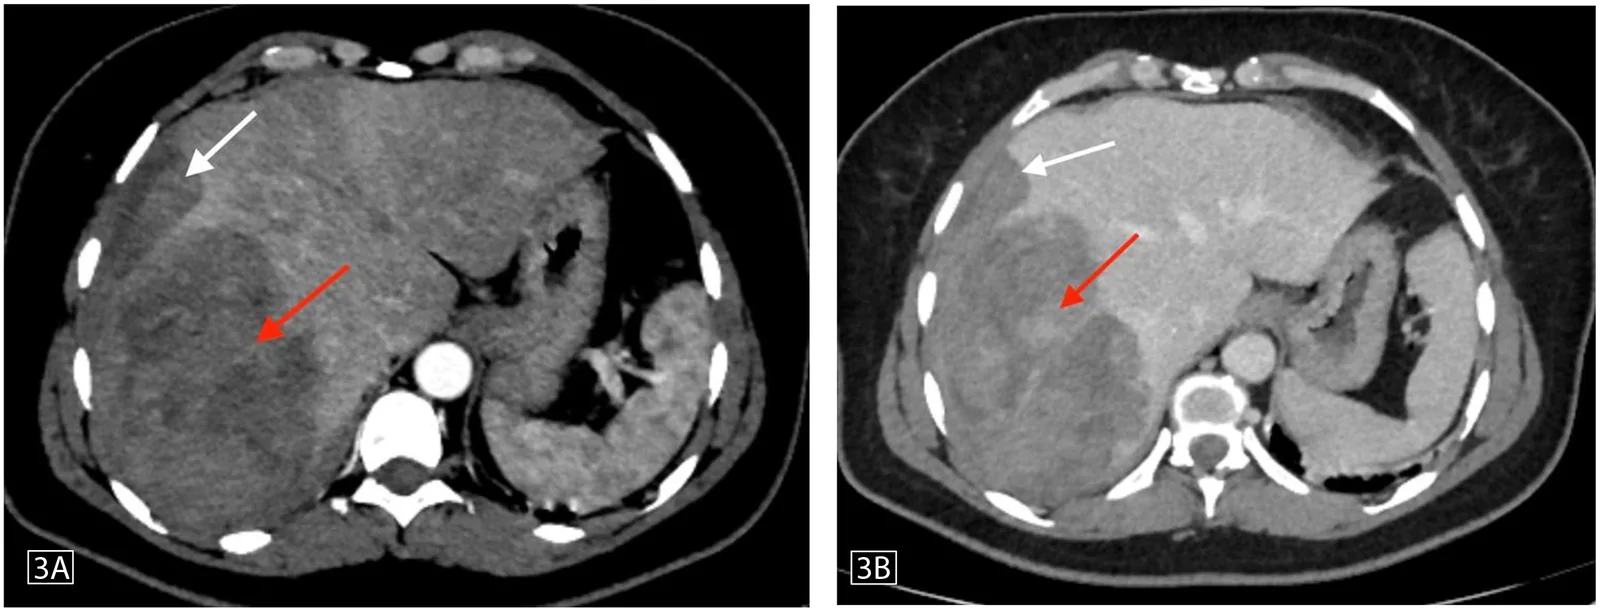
Contrast-enhanced CT appearance of the largest hemorrhagic hepatocellular adenoma. (A and B) Arterial and portal-phase contrast-enhanced CT images showing the large hemorrhagic lesion involving segments VII and VIII (red arrow) and the associated subcapsular hematoma (white arrow). The lesion and hematoma remain hypoattenuating relative to the surrounding liver parenchyma, without appreciable enhancement or definite active contrast extravasation.
